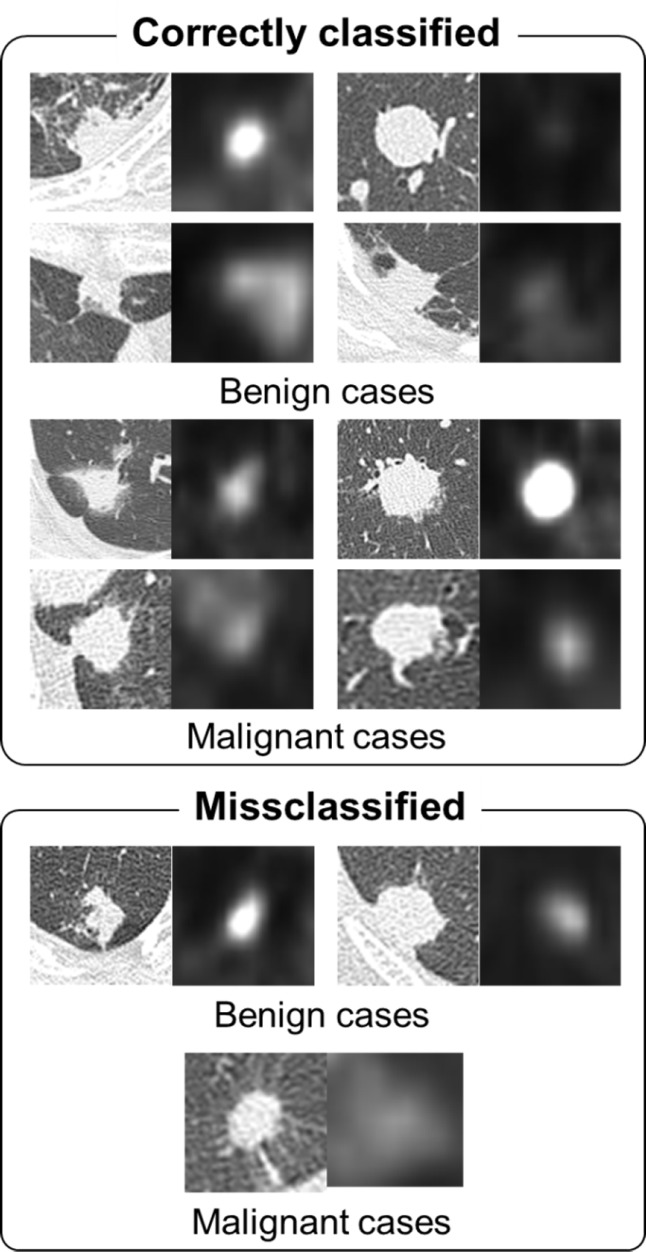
classification results(Images on the left are CT images and images on the right are early phase PET images. The two values under the images are the SUVs of early and delayed PET images).
